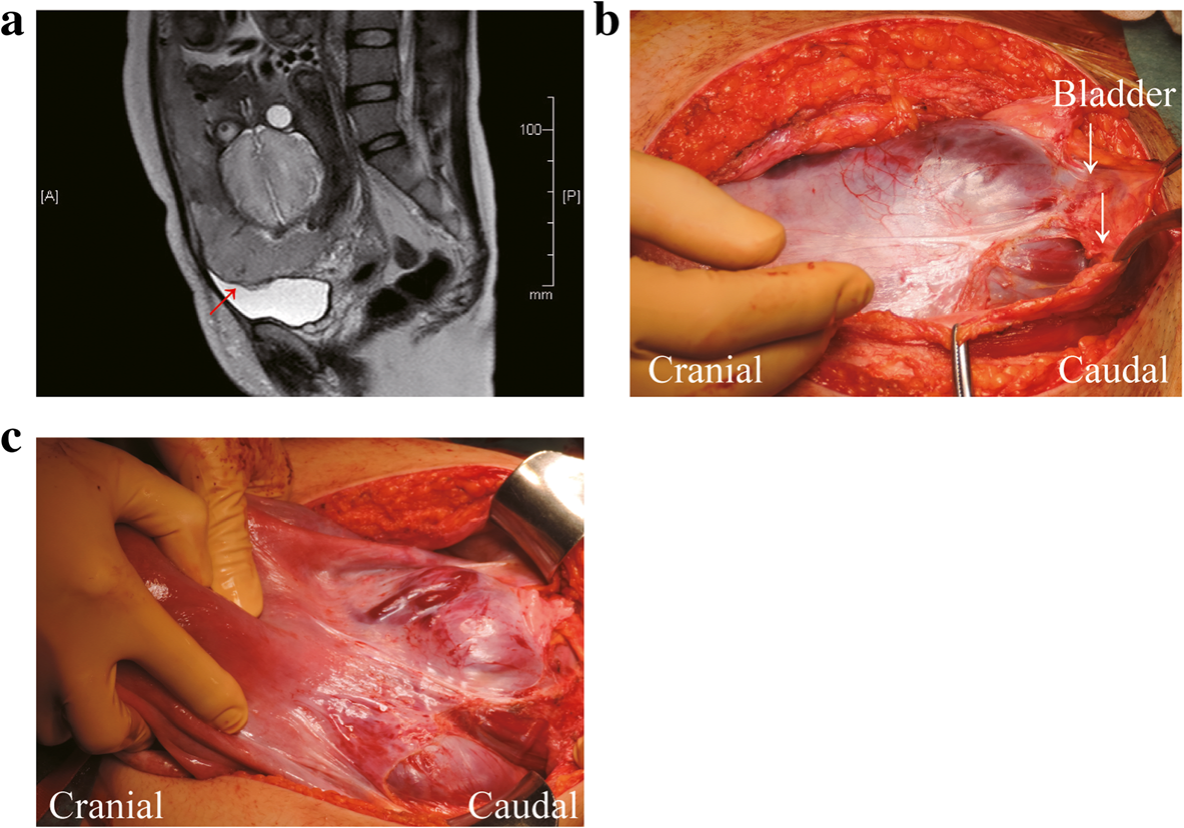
a A pelvic MRI was performed at 35 weeks of gestation. Red arrow indicates the loss of uterine myometrium between the placenta and the bladder wall. Based these findings, we suspected placenta percreta with bladder involvement. b and c, intraoperative findings revealed that placenta percreta involved the bladder and approached the pelvic sidewall and filled the cul de sac. White arrow indicates strong adhesion between the placenta and bladder
